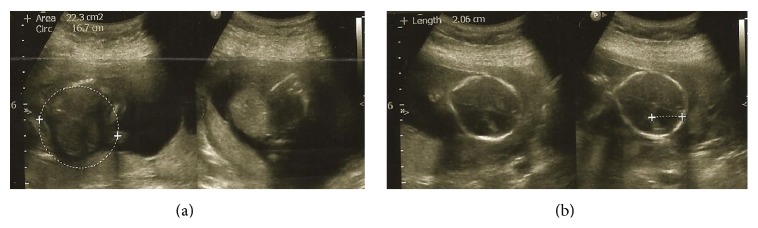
The image of the prenatal ultrasound scan. (a) The omphalocele sac (arrowhead) containing the liver and bowels. (b) The lemon sign (arrowhead).
